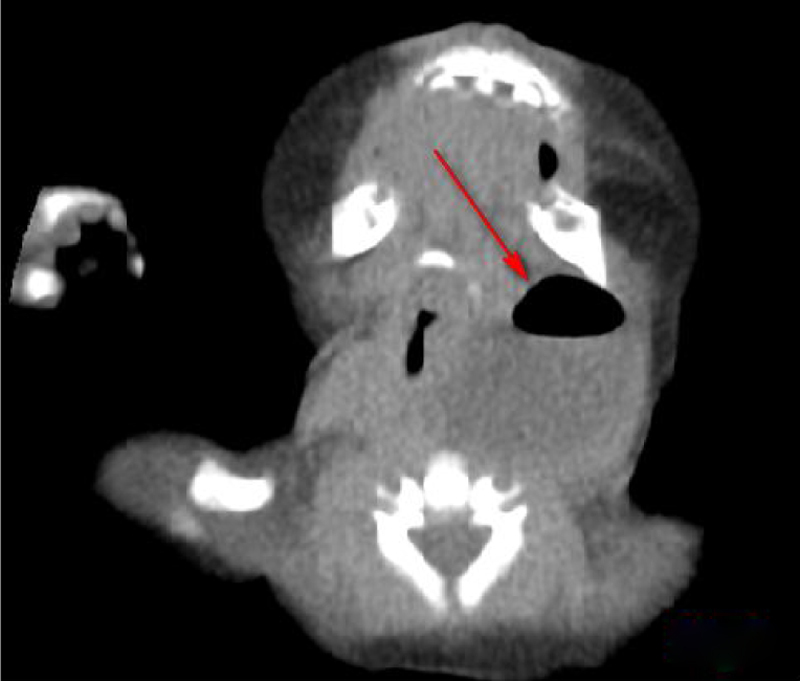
Enhanced CT: Parapharyngeal space abscess and air shadow (Horizontal scan).
